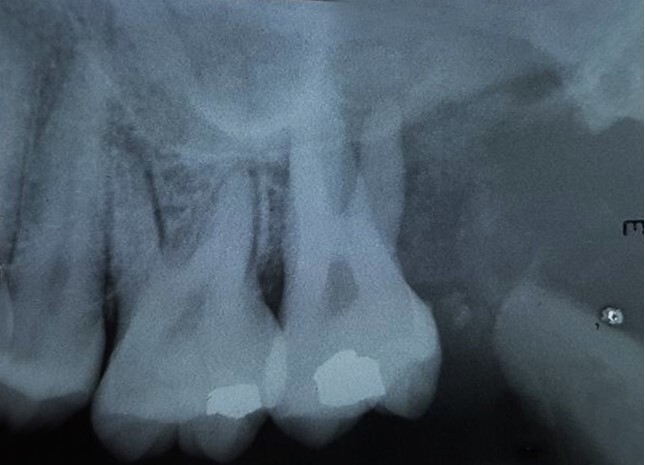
Periapical radiograph, showing bone destruction in the area of the absent third molar and around the second molar, reaching the maxillary sinus, and widening of the space occupied by the periodontal ligament around the first molar.
